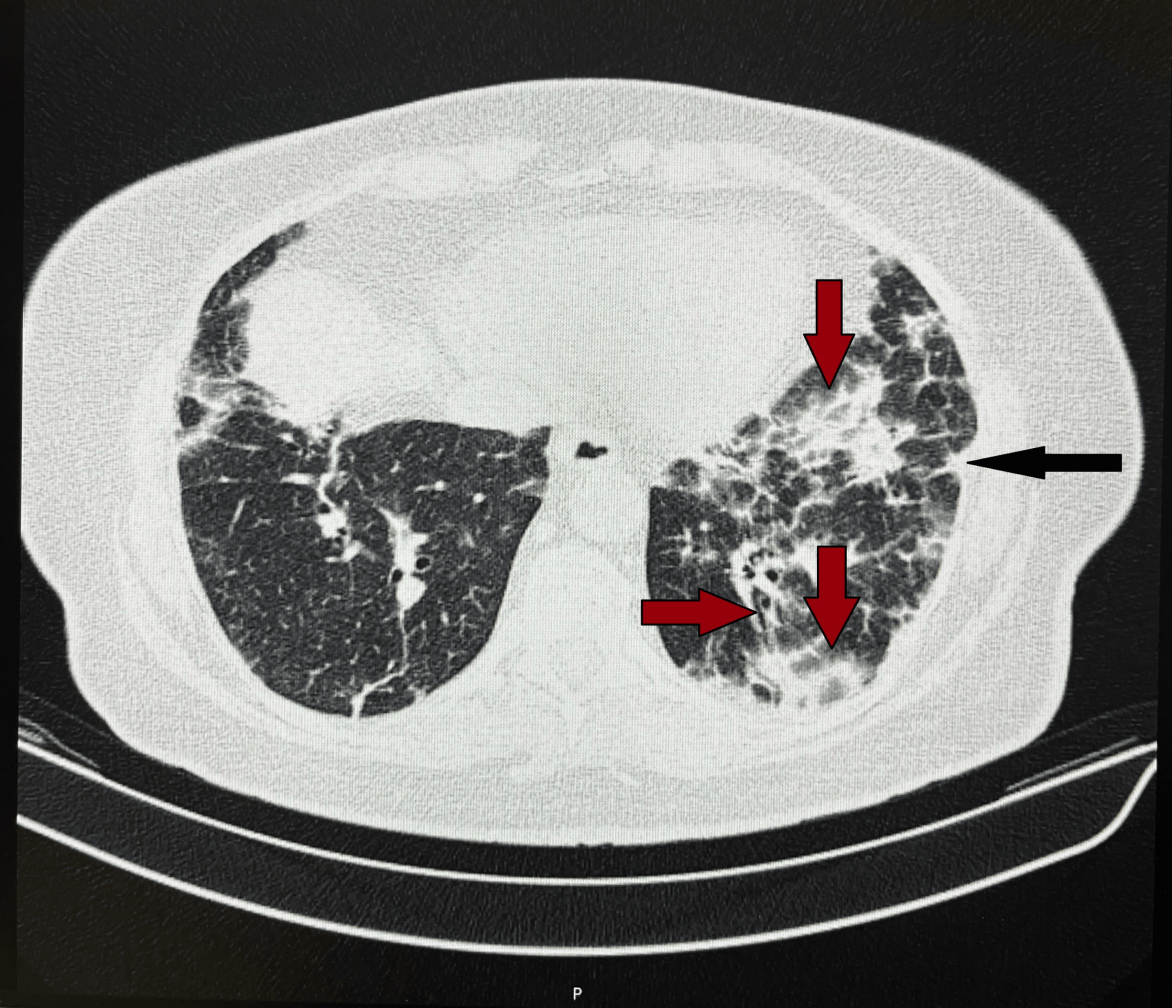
First lung CT scan (axial view) CT: Computed Tomography The black arrow shows subpleural consolidation in the left lung; the red arrows show ground-glass opacities and bronchiectasis in the left lung.
